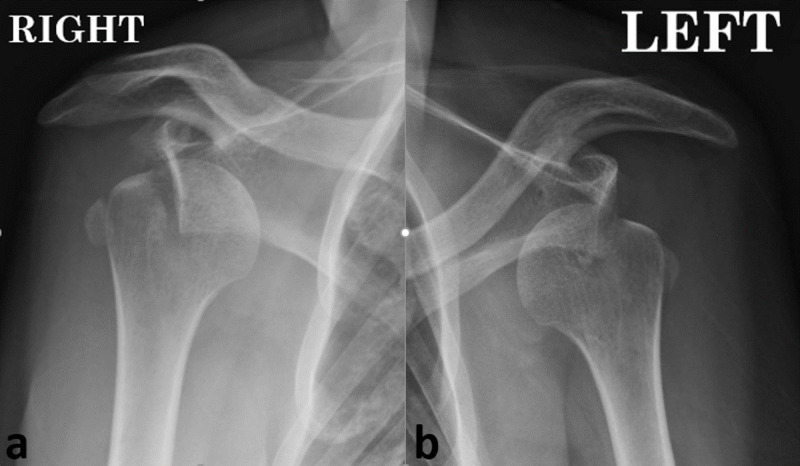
(a, b) Preoperative antero-posterior radiographs
